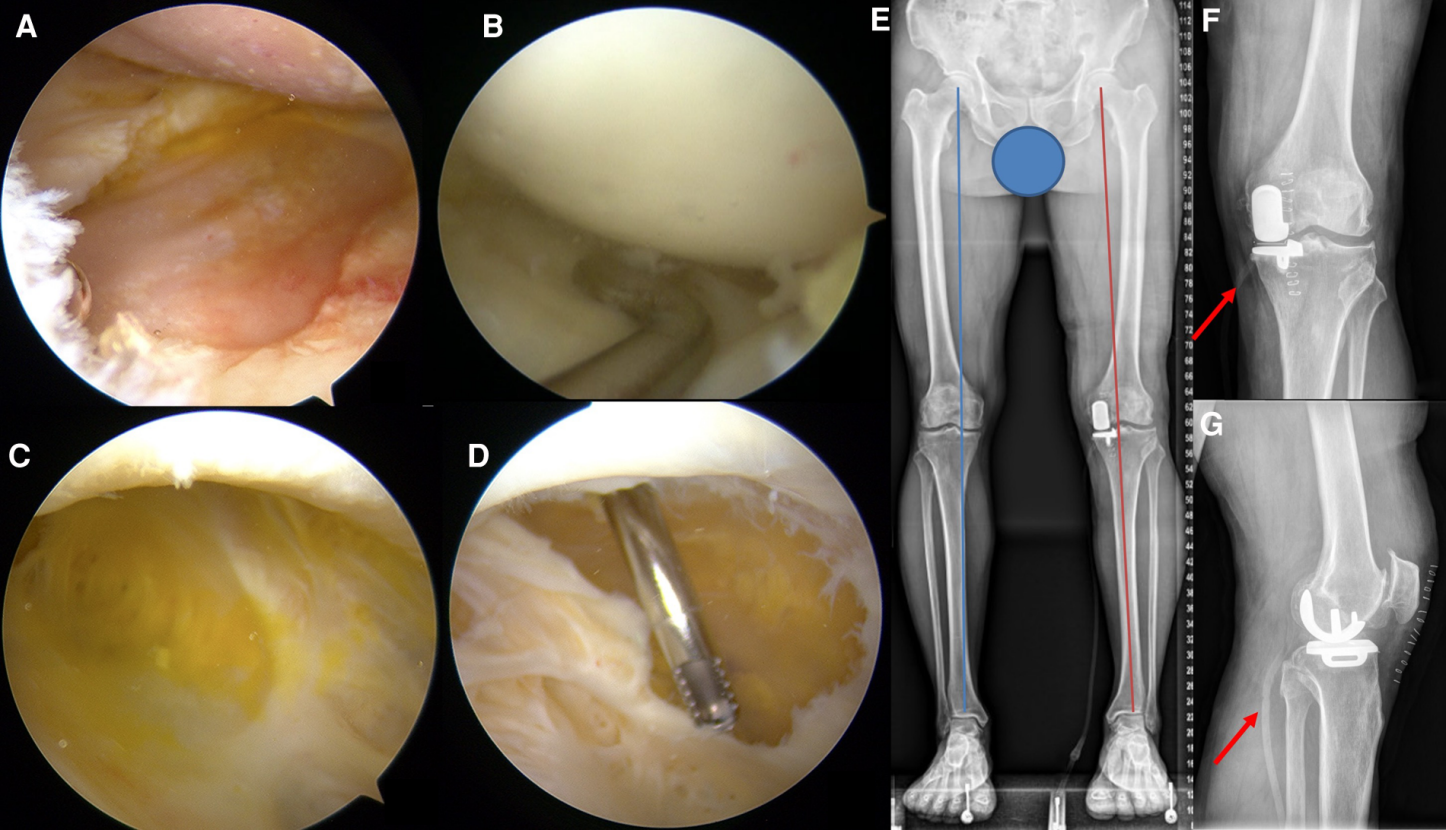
Arthroscopic surgery view (A–D) and immediate postoperative X-ray (E–G) evaluation after the surgery. (A) Severe cartilage wearing of the medial femoral and tibial condyles. (B) Normal lateral meniscus and cartilage of the femoral and tibial condyles. (C) The wall of the popliteal cyst is filled with orange juice-like fluid. (D) Resection of the cyst wall. (E) The Weight-Bearing line (red) of the left lower extremity returned to a normal position similar to the contralateral side (blue). (F,G) Long red arrows indicated the drainage tube.
